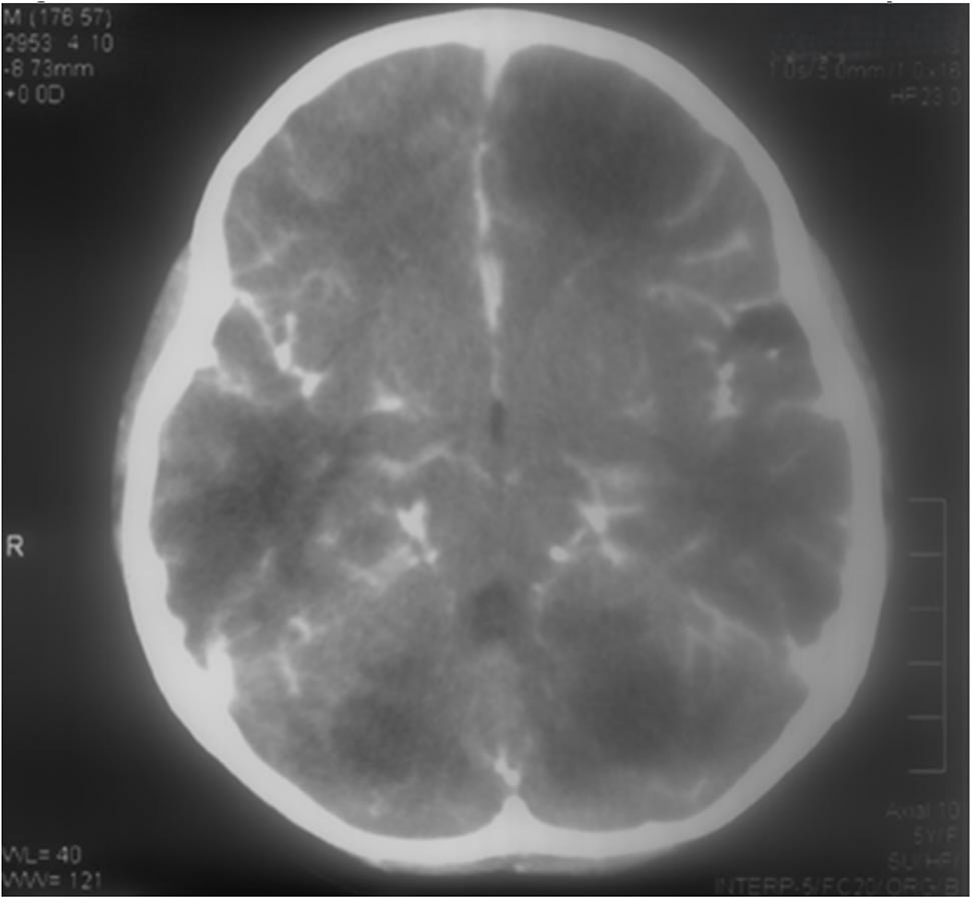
Contrast Brain CT Scan of B. mandrillaris encephalitis patient
